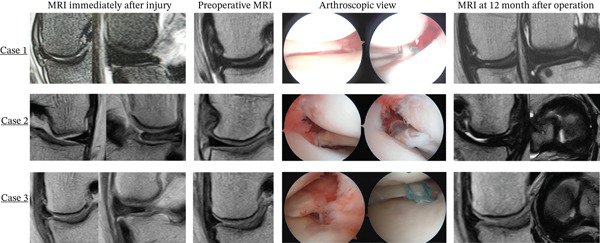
MRI and arthroscopic findings. Left: MRI immediately after injury showed MCL injury and MMPRTs. Middle: Preoperative MRI showed the progression of extrusion of the medial meniscus. Arthroscopic view showed the MMPRTs (left) and the image after the repair (right). Right: MRI at 12 months after operation showed the posterior root repair and the improvement of extrusion of the medial meniscus.
